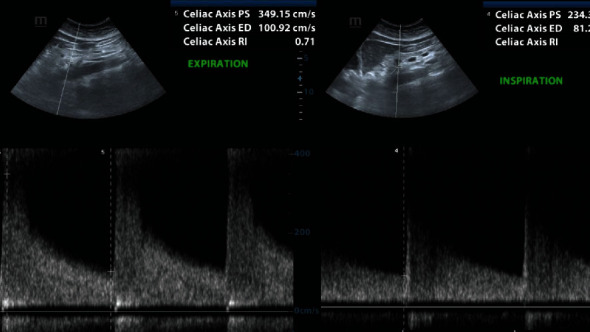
Duplex Doppler ultrasound images demonstrating narrowing of the CMT during forced expiration and dilation in the inspiratory phase. A maximum expiratory peak systolic velocity of 374 cm/sec was recorded within the coeliac axis.
